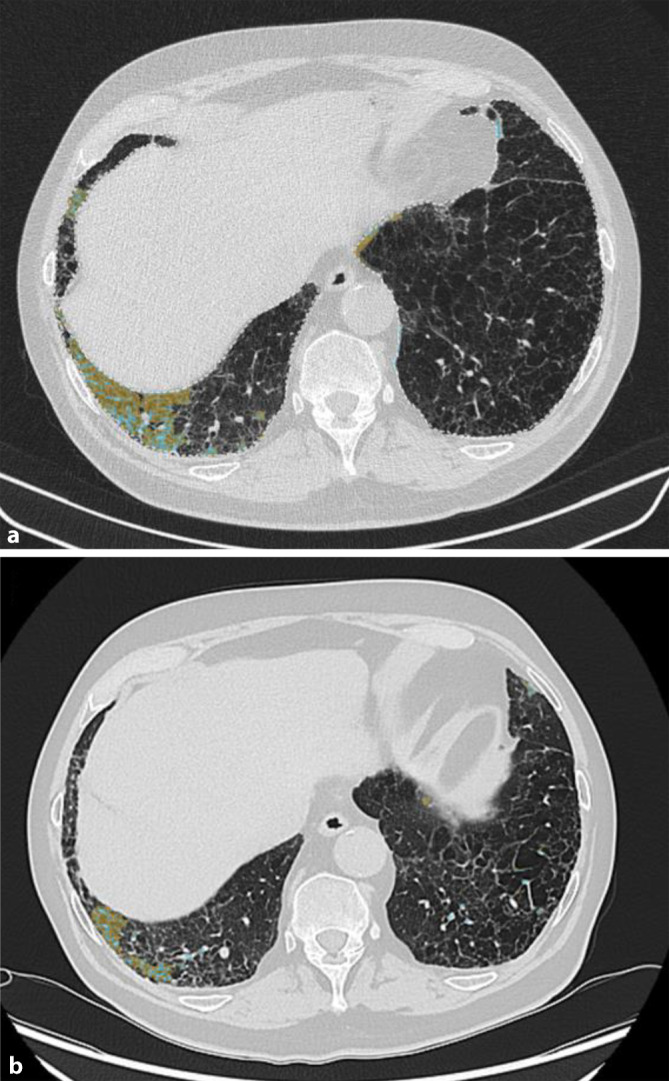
Künstliche Intelligenz basierende Quantifizierung der pulmonalen hochauflösenden Computertomographie („artificial intelligence-based quantification of pulmonary HRCT“ [AIqpHRCT]) der Milchglastrübungen a Baseline: 1,2 % und b Monat 6: 0,8 %
